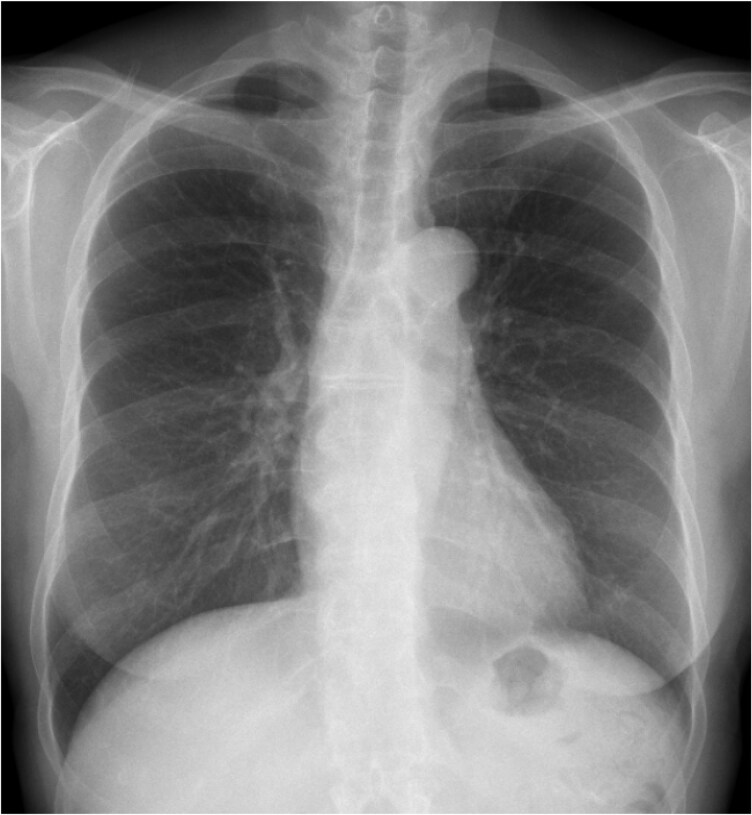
Chest radiography showed no signs of pulmonary congestion or enlargement of the heart shadow.
